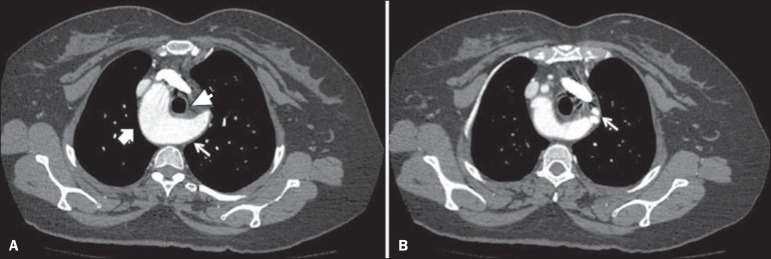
Computed tomography angiography of the chest, in the axial plane.
